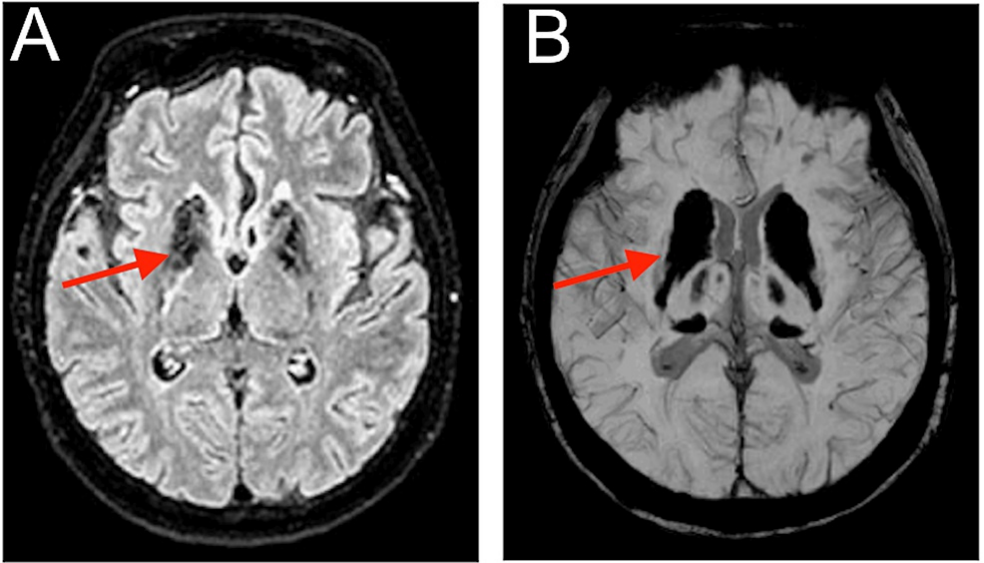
Brain MRI. Brain MRI demonstrating T2 hypointensities and blooming artifacts in these regions (A-B, arrows). These findings along with the head CT were consistent with diffuse brain calcifications.
